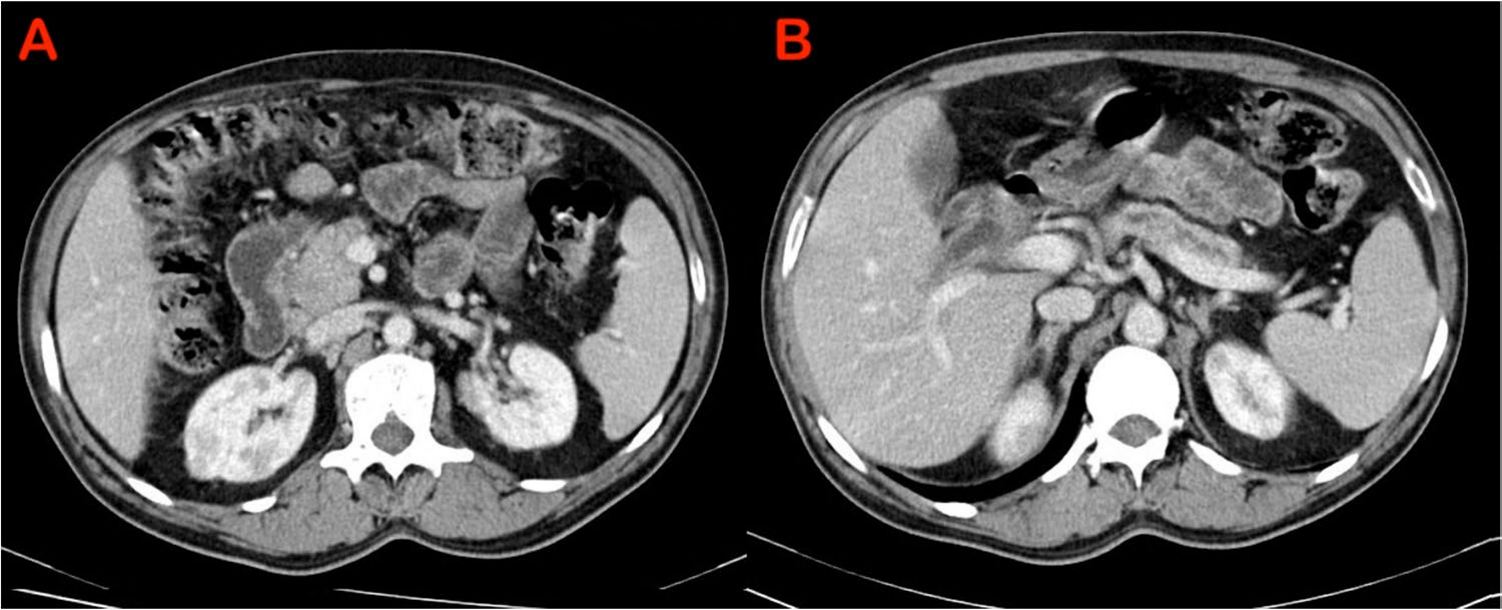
Computerised tomography (CT) shows (A) focal enlargement of the head of pancreas (B) mildly dilated pancreatic duct in body and tail - suggestive of focal autoimmune pancreatitis
